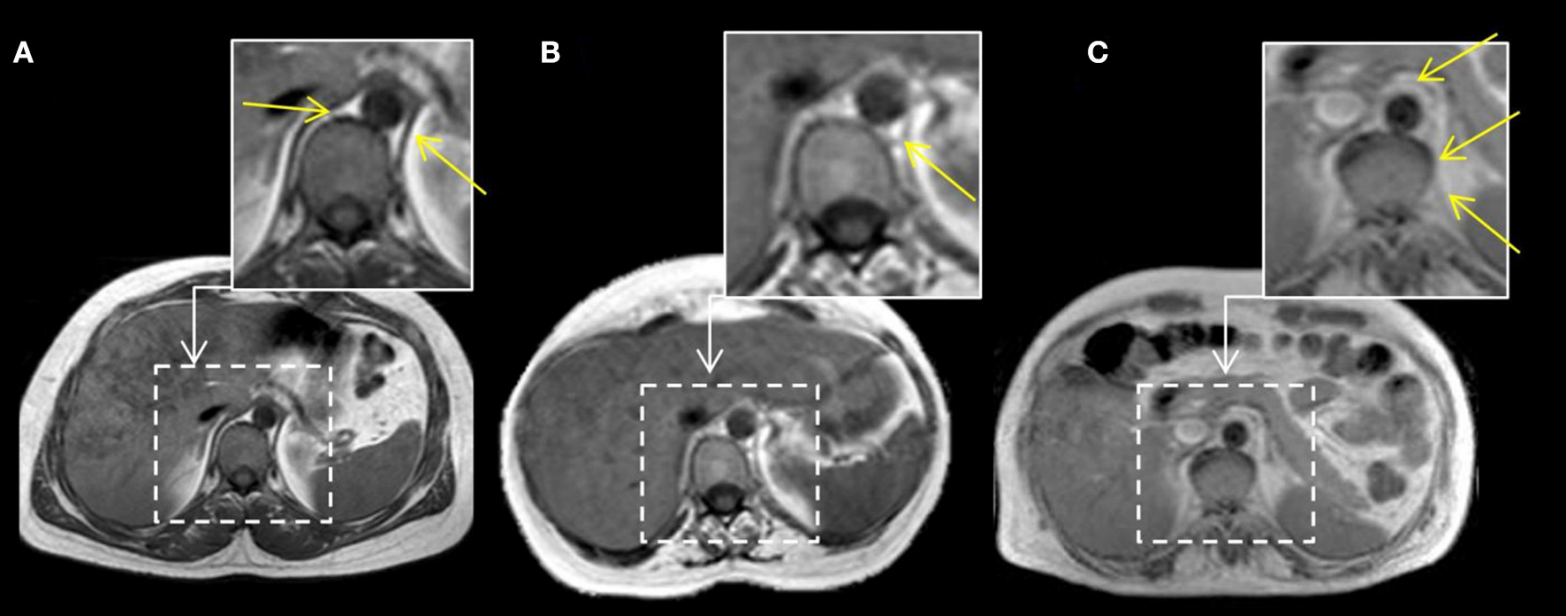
MRI T1w abdominal sections of three LOPD patients. Yellow arrows point to both diaphragm pillars. In case (A), right and left diaphragm pillars are visible and do not show fatty replacement (Mercuri score 0). In case (B), left diaphragm pillar is visible and partially infiltrated, whereas right diaphragm pillar is absent (left pillar Mercuri score 3 points). In case (C), we can observe fully replaced diaphragm pillars at both sides (Mercuri score 4 points).
